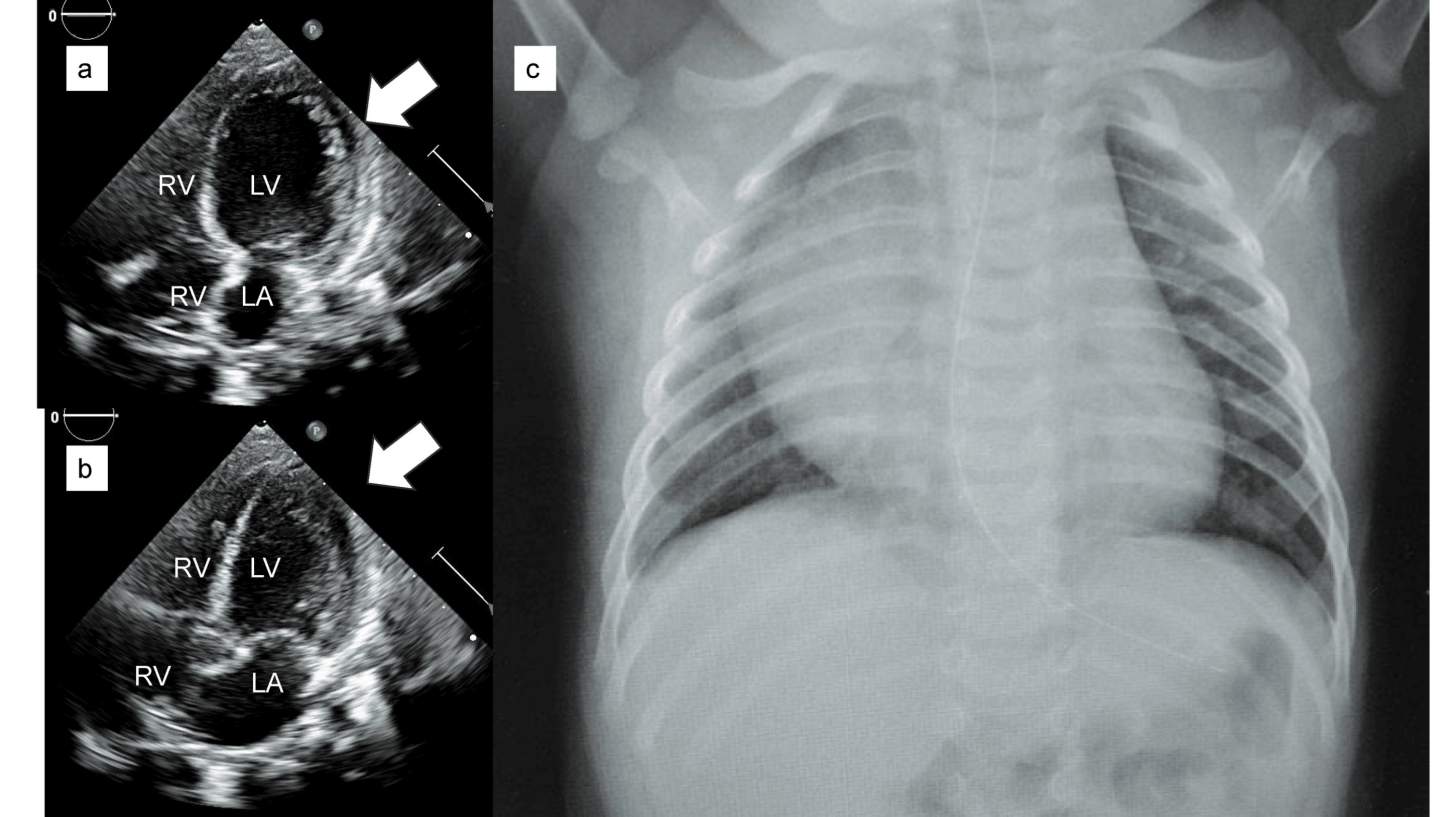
Transthoracic echocardiography and chest radiograph at five months. Echocardiography reveals no obvious left ventricular (LV, white arrowhead) enlargement, myocardial hypertrophy, or wall-motion abnormalities in four-chamber views during (a) diastole and (b) systole. (c) The chest radiograph shows no cardiac enlargement, with a cardiothoracic ratio of 55%. RV, right ventricle; RA, right atrium; LA, left atrium
